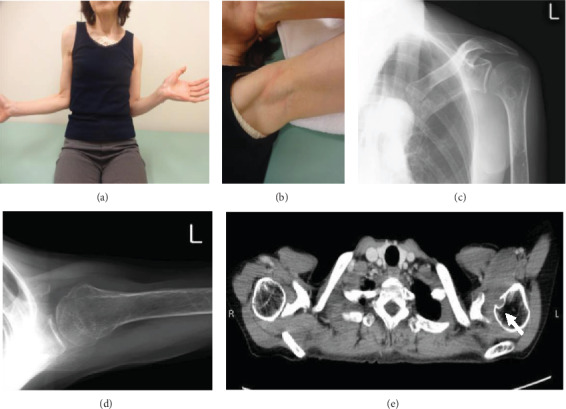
(a, b) Photograph of the patient at her first visit, with maximal external rotation of the shoulder (a), and photograph of the left axillary lump (b). Anteroposterior (c) and lateral (d) views on plain X-ray show a well-circumscribed, oval, osteolytic lesion with sclerotic margins in the lesser tubercle of the left humerus. (e) Contrast-enhanced computed tomography (CT) shows a soft tissue mass with invasion of the adjacent left humerus (arrow).
